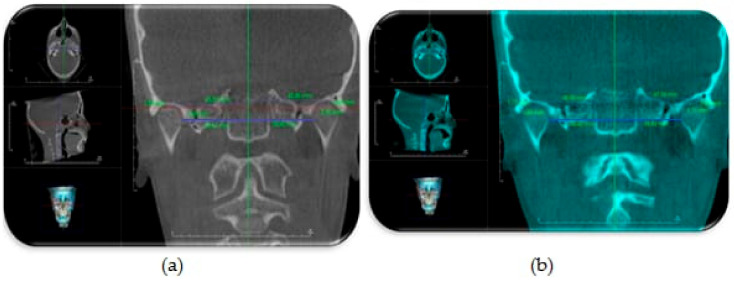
CBCT coronal generated view showing assessment of condylar head position relative to MSP (green line): (a) before TB therapy; (b) after TB therapy.
